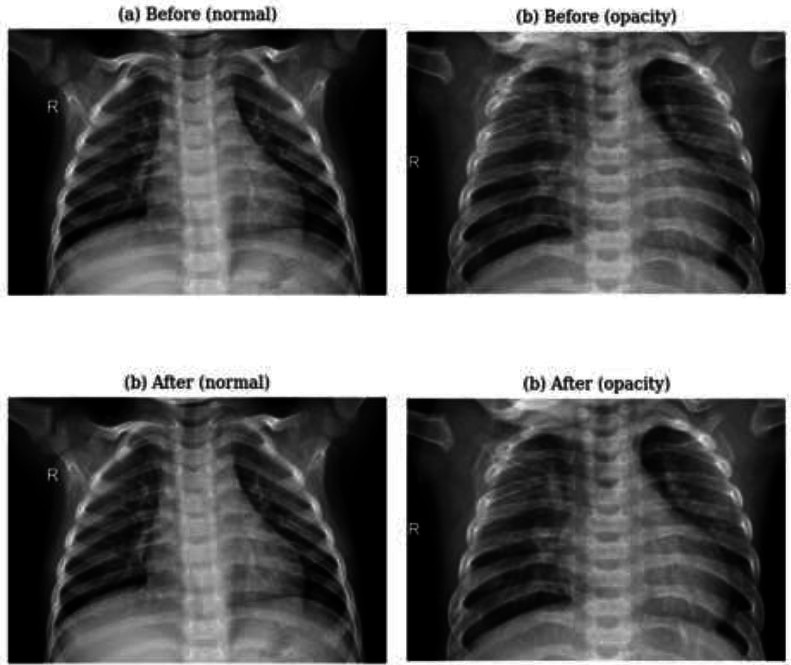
Chest x-ray images of normal cases and pneumonia cases before and after image preprocessing.
